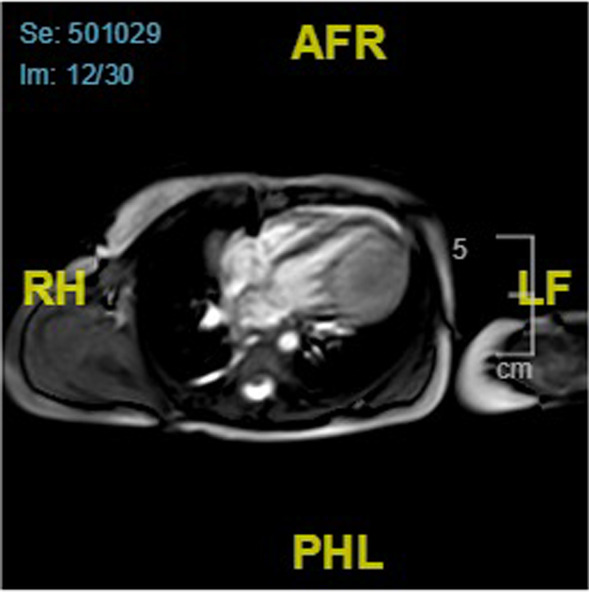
Cardiac magnetic resonance imaging girl. Legend: Cardiac magnetic resonance imaging showing an intracardiac iso- to hypointense mass in the left ventricle in the native T1w-weighted sequence
